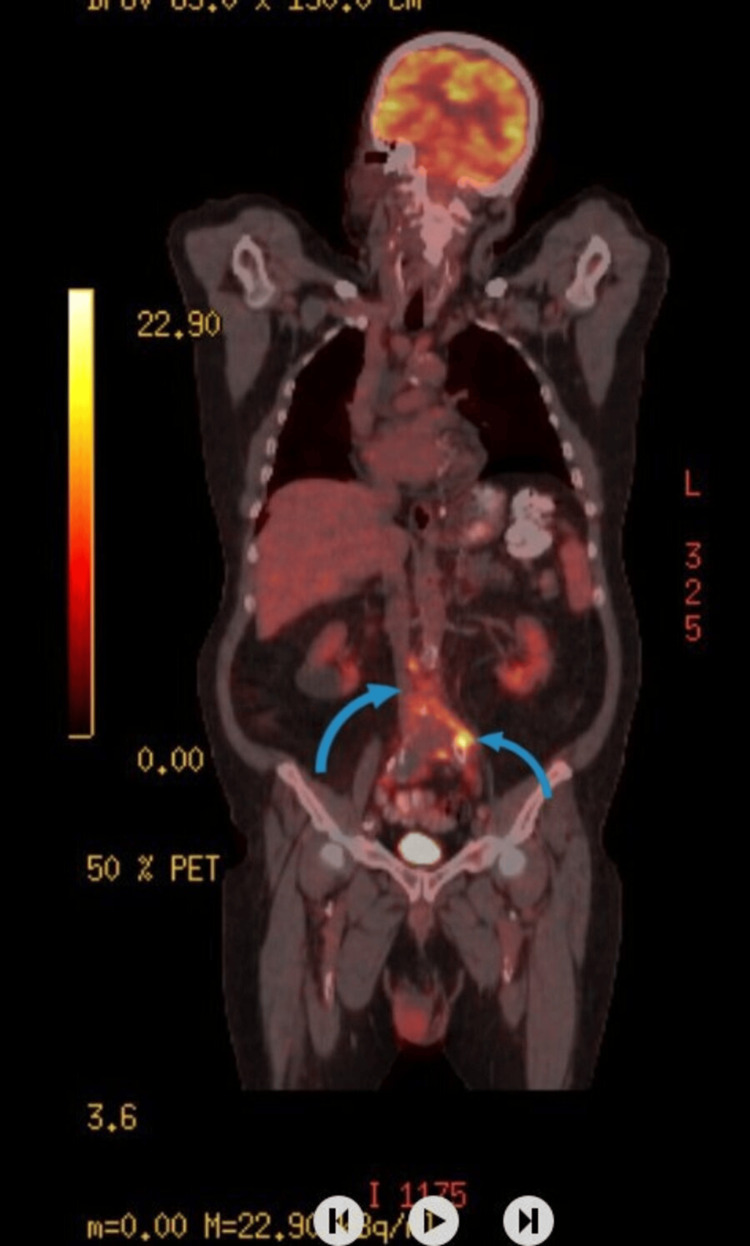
A full-body 18F-fluorodeoxyglucose (18F-FDG) PET-CT revealed increased perivascular thickening and FDG uptake (maximum standard unit value (SUVmax) 9.17) along the distal abdominal aorta and common iliac arteries, with inflammatory involvement of the anterior L4-L5 vertebrae and disc. A hypermetabolic (SUVmax 7.51) 10 mm inter-aortocaval lymph node was also detected.
